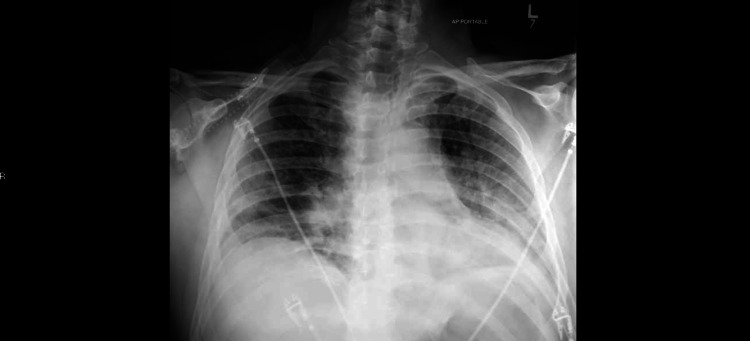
Chest X-Ray on the day of admission
